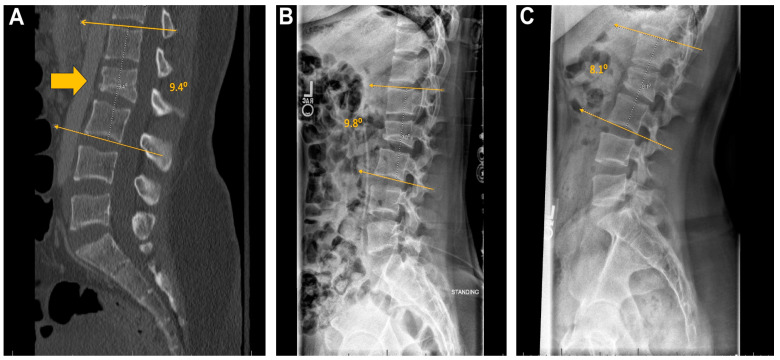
Example spinal alignment assessment in a patient managed with a brace. (A) CT-Lumbar spine with para-sagittal view demonstrated fracture morphology (arrow) at L2. Standing X-rays with lateral views at post-injury with brace (B) day 5, and (C) ~3-months.
